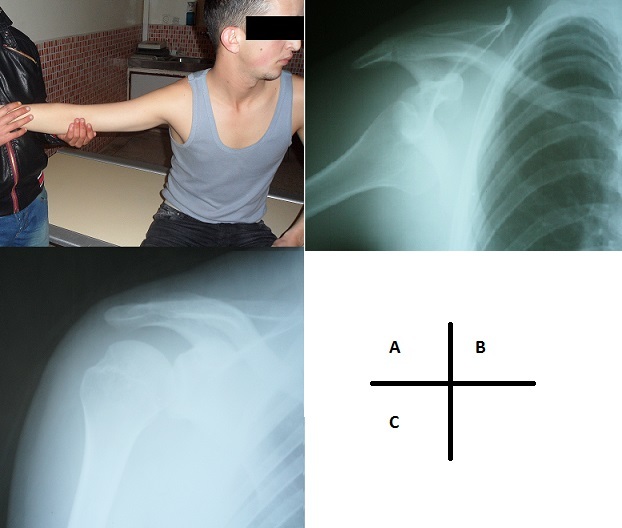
A) Position du patient aux urgences; B) Radiographie de l’épaule droite montrant une luxation erecta; C) Radiographie de contrôle après reduction
